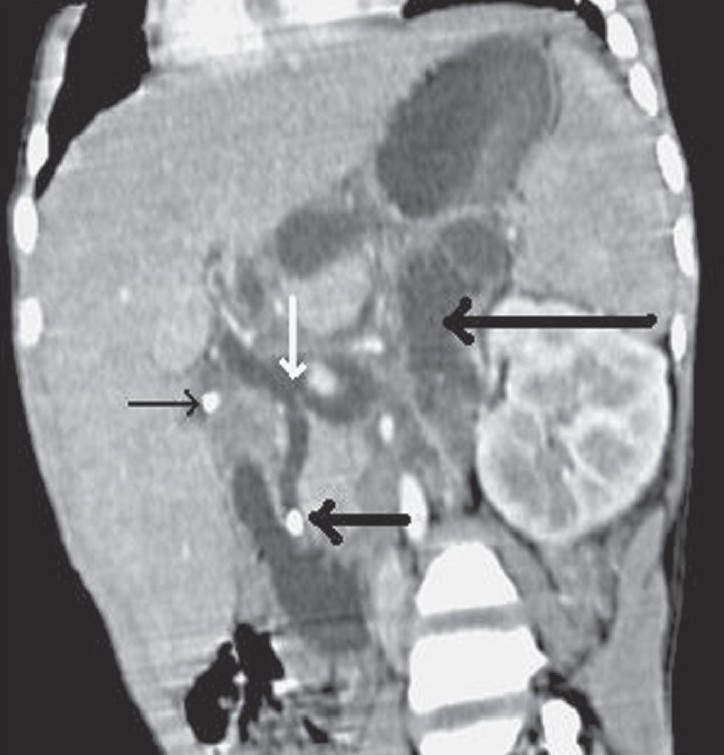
Oblique coronal MPR contrast-enhanced CT scan shows a calculus in the distal part of the CBD (thick black arrow), with proximal dilatation and a calculus in the neck of gallbladder (thin black arrow). In addition there is frank communication (white arrow) between the extrahepatic suprapancreatic CBD and retroperitoneal collections, lying between the left kidney and the pancreas and pushing the stomach superiorly (large black arrow)
